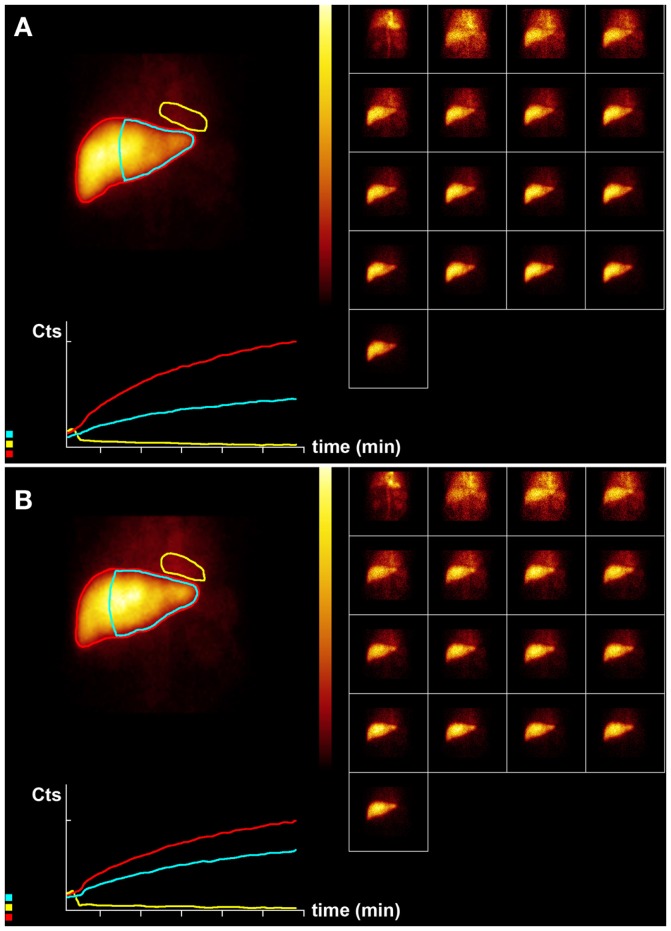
Hepatobiliary scintigraphy before (A) and 6 weeks after (B) whole liver treatment with 1.7 GBq ^90^ Y labeled SIR-Spheres in a 65-year-old male patient with multifocal predominantly right-sided recurrent hepatocellular carcinoma (Figure 1). Displayed are summed dynamic frames (2 × 10 s/frame), a summed image (20 × 10 s) with regions of interest drawn around the entire liver (red), left liver segments 2–4 (blue), and cardiac blood pool (yellow), and corresponding time–activity curves. The total liver function (body surface area corrected mebrofenin uptake rate) was reduced after treatment from 7.4 to 6.1%/min (red curves). However, function of left liver lobes 2–4 increased from 3.4 to 4.1%/min (blue). This functional increase is both relative and absolute and can be explained by insufficient post treatment functional liver capacity leading to functional hypertrophy in non-affected liver segments.
